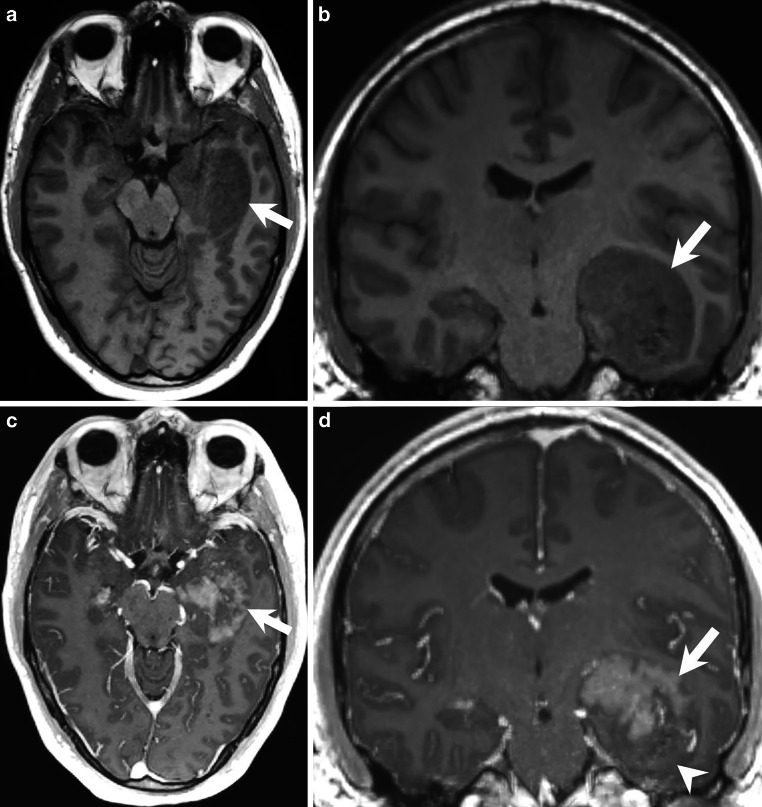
On axial (a) and coronal (b) T1-weighted images, the lesion appeared homogeneously hypointense (a, b, *arrows*). On axial (c) and coronal (d) T1-weighted images taken after administration of gadolinium (Gd), the lesion showed large areas, predominantly in the upper part of the lesion, with clear and homogeneous but not pronounced enhancement (c, d, *arrows*). In particular, the lower part of the lesion showed no contrast enhancement (d, *arrowhead*)
